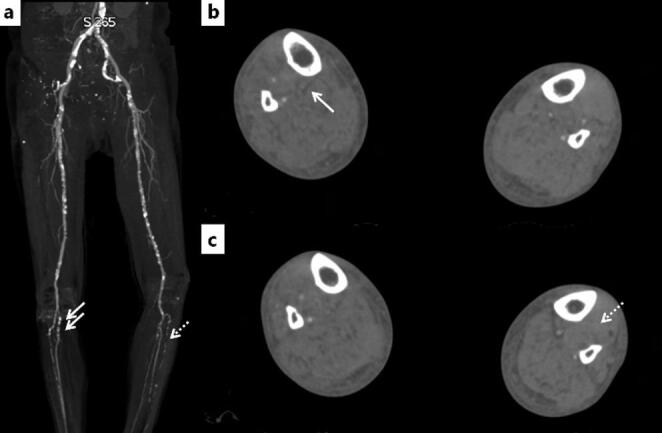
Peripheral artery disease (PAD). a CTA, b–c axial CT. A woman in her 70s with type 2 DM presented with numbness and pain in both lower extremities. MIP image from peripheral CTA demonstrates extensive atherosclerotic disease with multifocal stenosis/occlusion. The right posterior tibial artery is occluded (arrow) and the proximal left anterior tibial artery is occluded (dotted arrow). Abbreviations: DM, diabetes mellitus; MIP, maximum-intensity projection; CTA, computed tomography angiography
